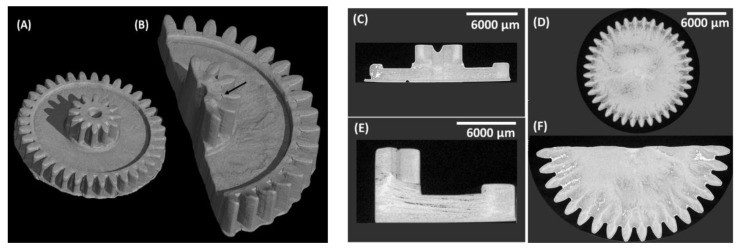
µ-CT images of the gear-shaped objects fabricated from 55BM_80_PI_1_D_0.015_E_10_ (A,C,D) and 60BM_80_PI_1_D_0.015_E_10_ (B,E,F) resins. (C,E) are side views of (D,F).
